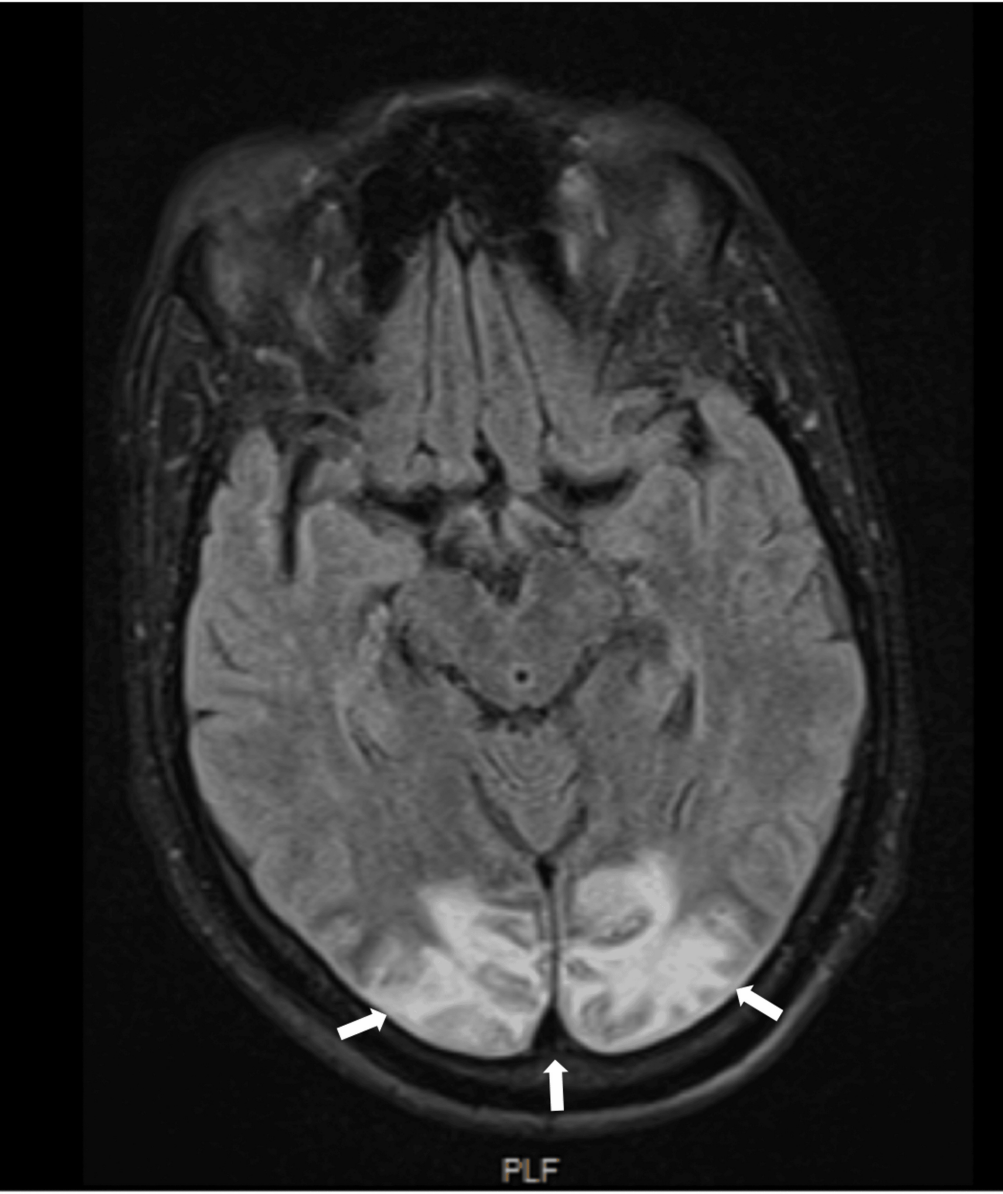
FLAIR sequence brain MRI, of the patient on postoperative day 5, showing areas of vasogenic edema in the parieto-occipital lobes FLAIR: Fluid-attenuated inversion recovery; MRI: Magnetic resonance imaging
